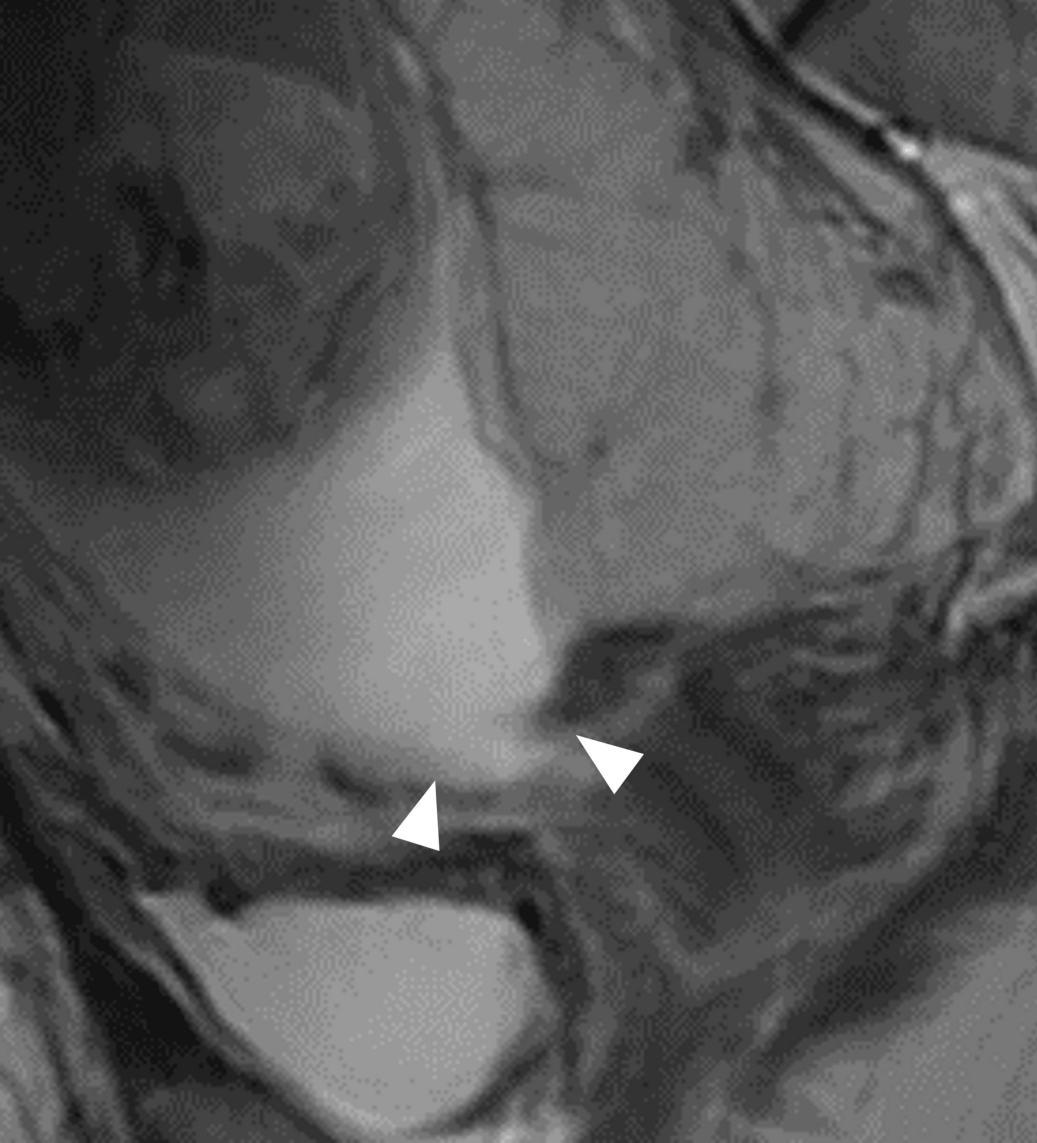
Sagittal T2WI MRI at 25 weeks gestation As in the ultrasound image, the decidual and chorionic tissues were thinning, and the boundaries were no longer discernible, but there was no evidence of fetal tissue bulging into the anterior vaginal fornix (arrowhead). T2WI: T2 weighted image, MRI: magnetic resonance imaging
